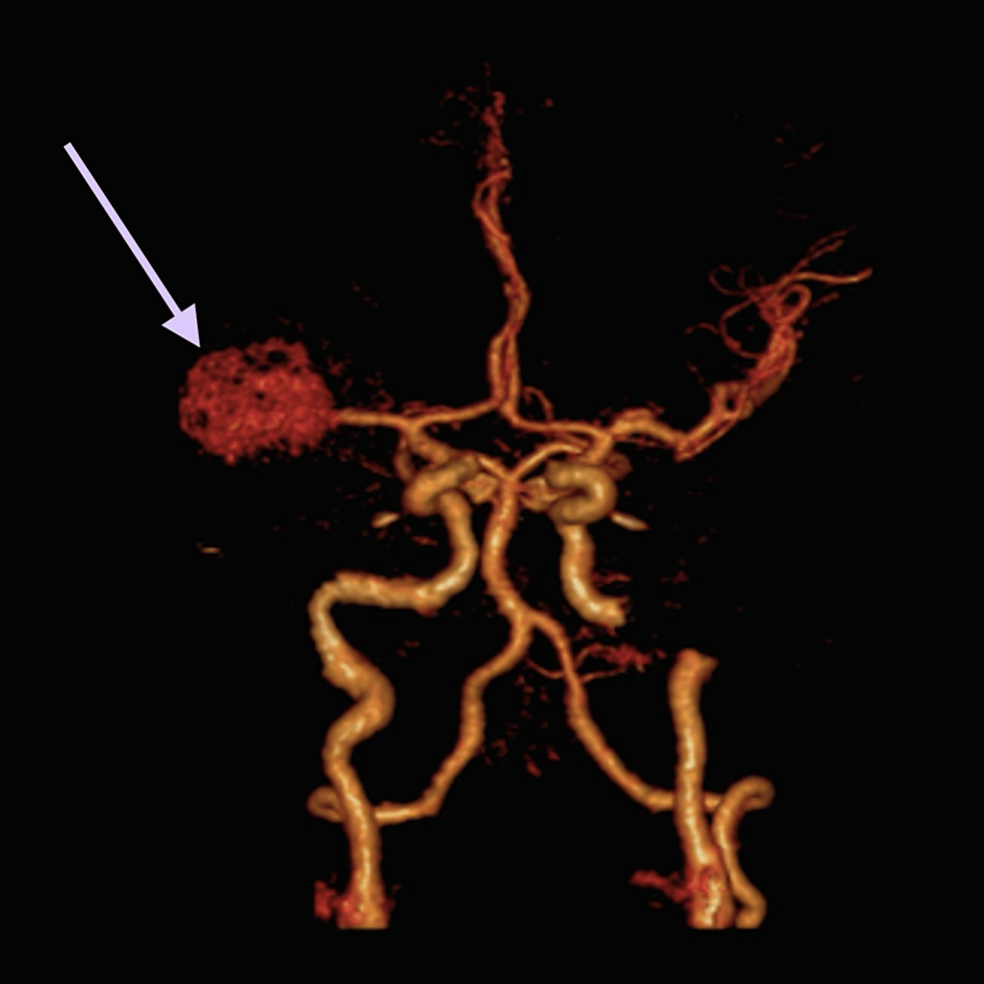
Angio CT on admission identified a giant aneurysm of the right middle cerebral artery.
